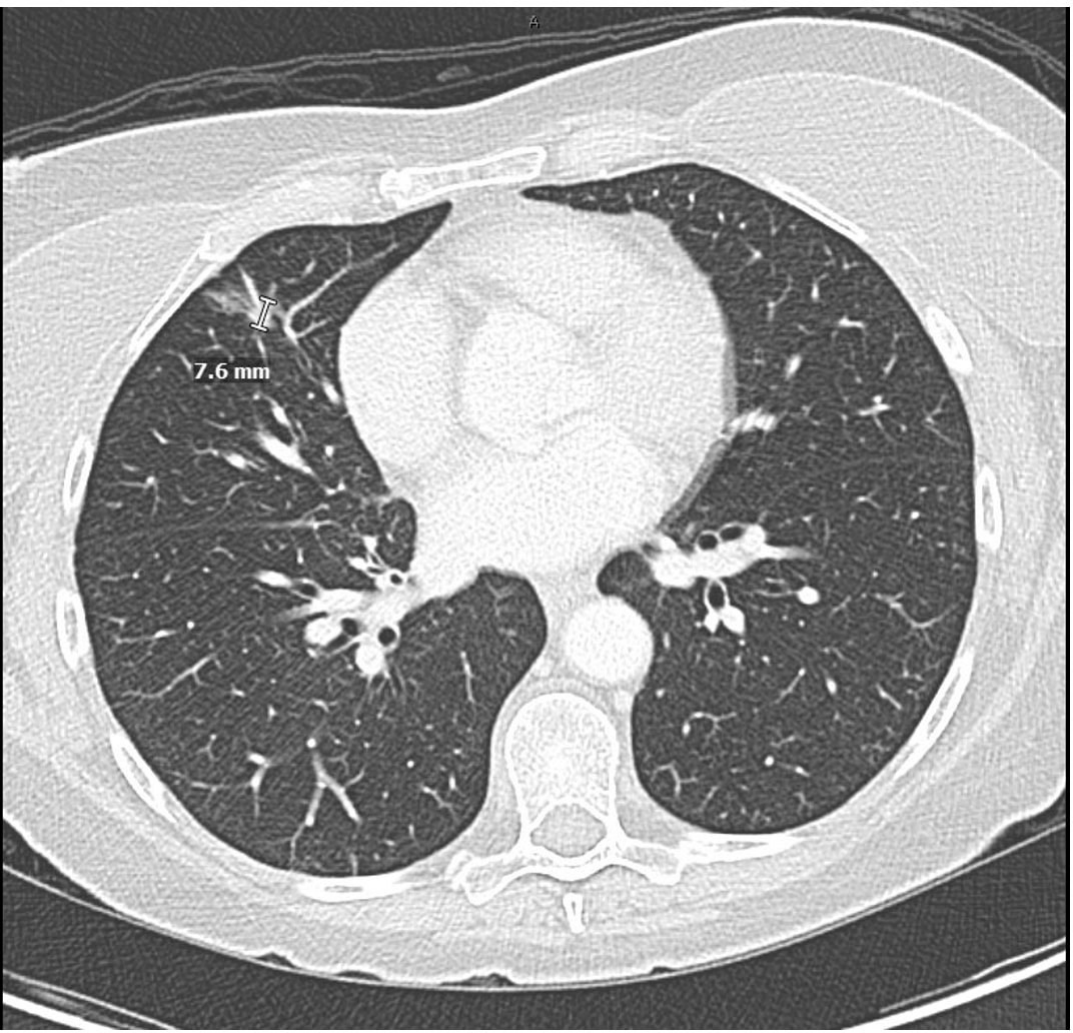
Chest CT with intravenous contrast 5 weeks after cessation of brigatinib demonstrating near-complete resolution of pneumonitis and resolution of right pleural effusion. Treatment response after 4 weeks of alectinib therapy showing decreased size of the primary right perihilar mass, decreased mediastinal and hilar lymphadenopathy, and improvement in metastatic disease.
